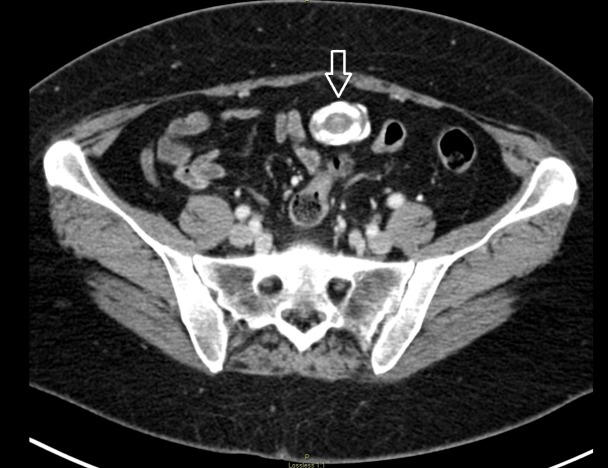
Computed tomography scan of abdomen and pelvis axial view showing calcified intra-abdominal mass (arrow).
